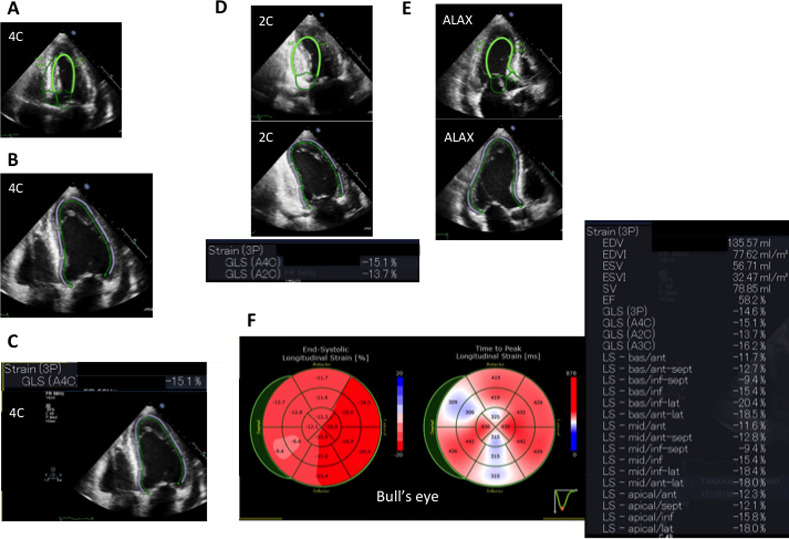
Step-by step approach to left ventricular (LV) volume and function measurements. A: Manual registration of apical 4-chamber view at end-diastole. B: Automatic LV endocardial border tracing (green line) at end-diastole in apical 4-chamber view. C: 2D speckle tracking analysis throughout the cardiac cycle, and a GLS of 4-chamber view was determined. D: The same procedures on apical 2-chamber view. E: The same procedures on long-axis view. F: Displayed bull’s eye, LV volumes, LV ejection fraction (EF) and global longitudinal strain (GLS).
